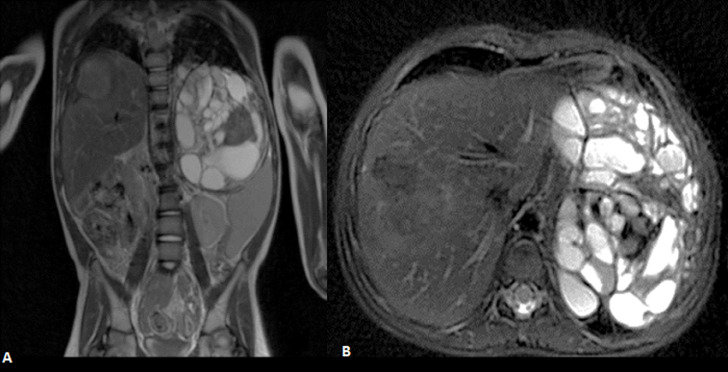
coronal (A) and axial (B) T2 weighted MR imaging showing a large left heterogeneous retroperitoneal mass with foci of calcification and fat. A large similar mass involving the VIII liver segment
